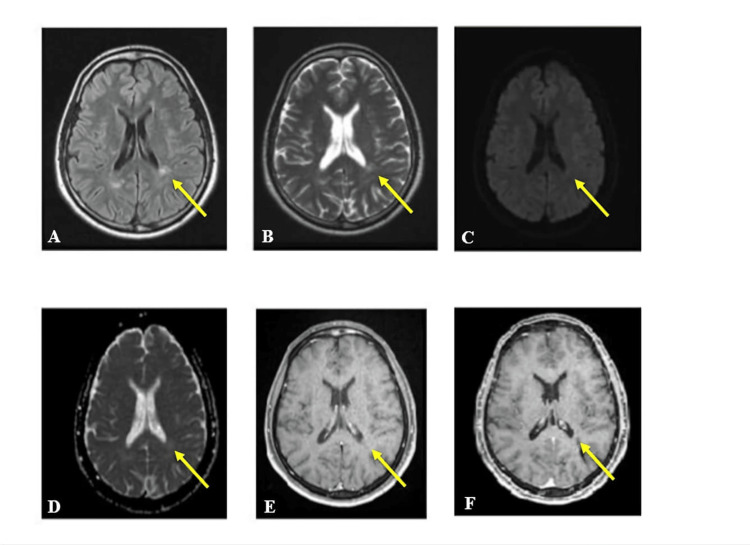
A 30-year-old male patient with inactive MS and subcortical and periventricular white matter lesions. (A) Axial FLAIR showed high signal intensity lesion (yellow arrow). (B) Axial T2 FSE high signal intensity for the same lesion. (C) DWI of the lesion was isointense. (D) ADC map of the same lesion was isointense. (E) Axial T1 FSE post-CE. (F) Axial MPRAGE delayed enhancement time. No enhancement for the lesion at both times. MS, multiple sclerosis; FLAIR, fluid attenuation inversion recovery; FSE, fast spin echo; DWI, diffusion-weighted image; ADC, apparent diffusion coefficient; CE, contrast enhancement; MPRAGE, magnetization-prepared rapid acquisition gradient echo
